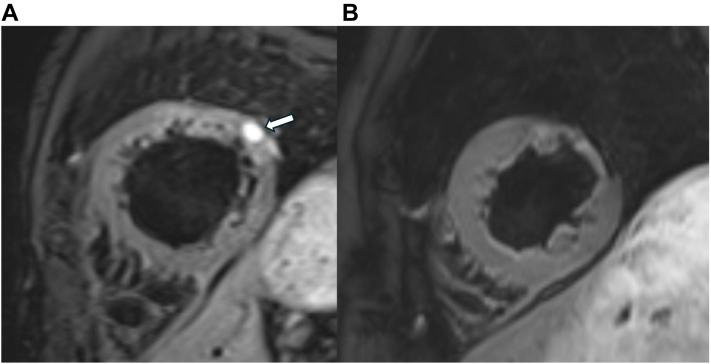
T2-Weighted Cardiac Magnetic Resonance Imaging (A) At 2 months: high signal intensity confirming organized thrombus. (B) At 21 months: complete resolution with left ventricular reverse remodeling. An arrow indicates the organized thrombus in A.
